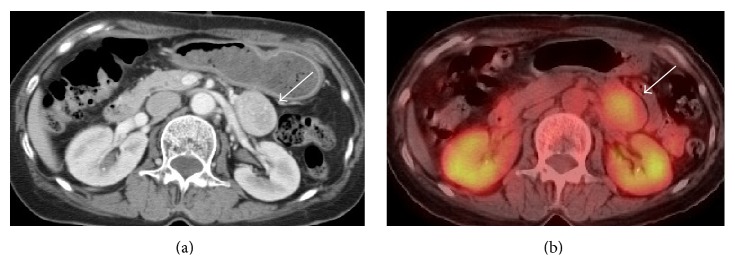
Abdominal mass (arrow). (a) CT revealed a 40 mm, well-circumscribed, firm, and well-enhanced mass adjacent to the upper jejunum. (b) PET-CT imaging showing the abdominal mass with an SUV max of 4.2.
